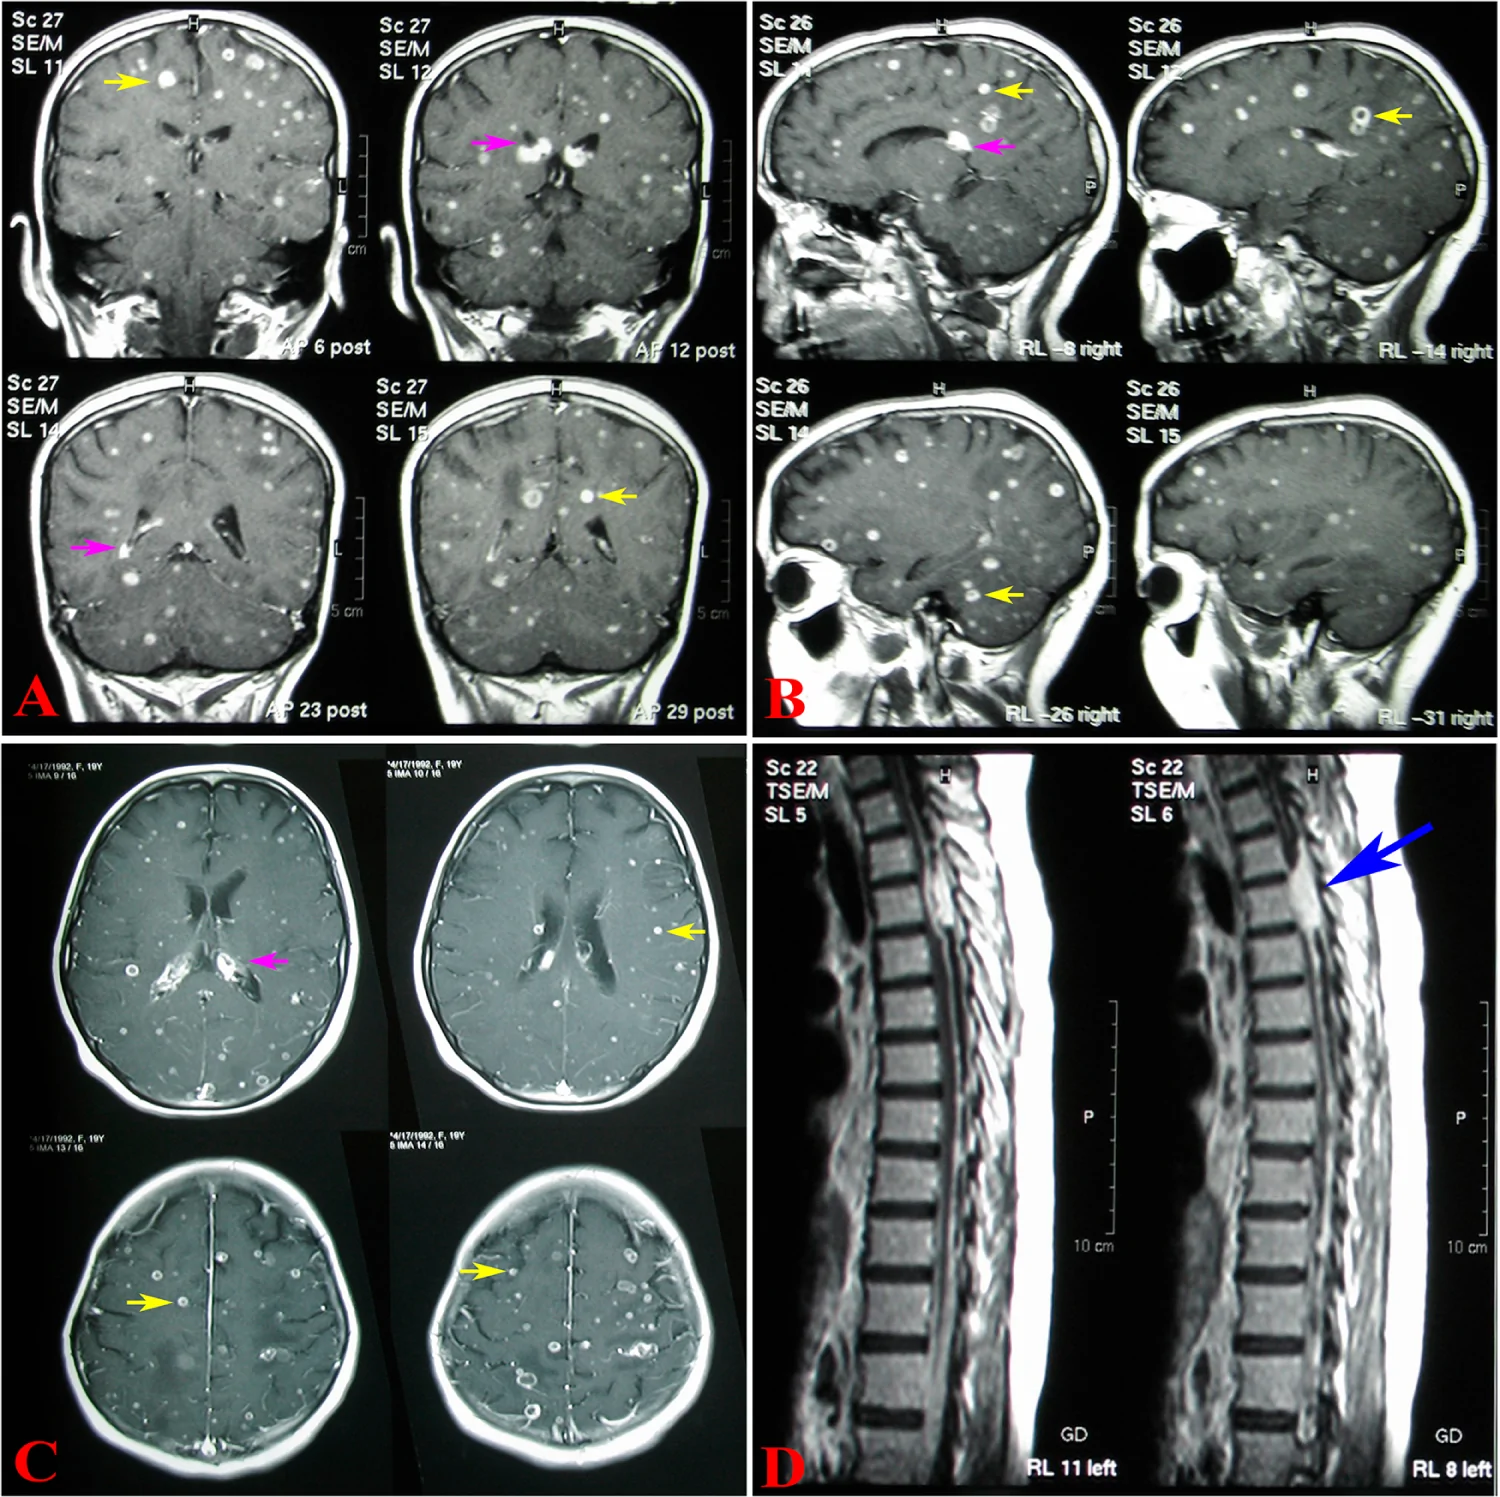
Multiple round hyper signal foci with peripheral enhancement and surrounding edema in the cerebral hemispheres and cerebellum (yellow arrows), compatible with tuberculoma; ependymal enhancement and ventricular sludge (magenta arrows), compatible with ventriculitis, in T1-weighted MRI: coronal (A), sagittal (B), and horizontal (C) views. Dural enhancement and hypersignal foci from C3 to C5 (blue arrow), compatible with intramedullary tuberculoma (D).
